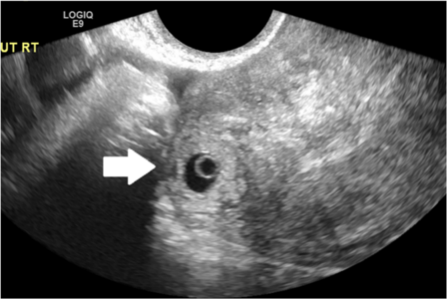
Interstitial ectopic pregnancy by transvaginal ultrasound. The arrow indicates thin (<5 mm) myometrium overlying the ectopic pregnancy. This finding by ultrasound, in combination with the lateral location of the gestation, has a reported specificity of 88-93 % but a sensitivity of just 40 % [101].
